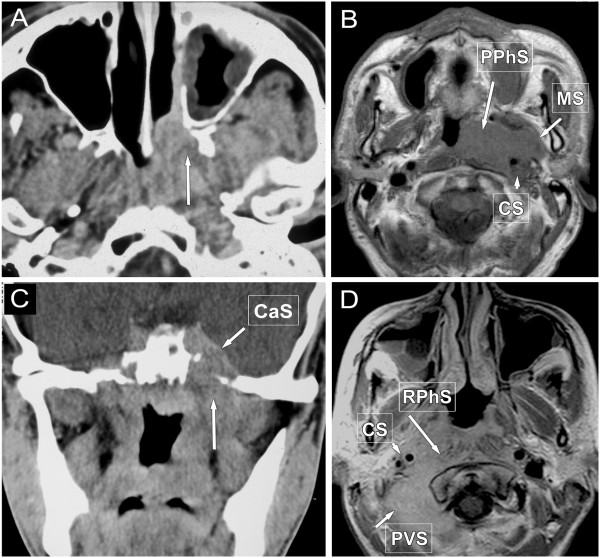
Different patterns of spread in nasopharyngeal cancer. A: Transverse post-contrast CT scan shows tumour destructing the left medial pterygoid lamina (long arrow), exceeding the choana and spreads along the lateral wall of the nasal cavity. Stage T3. B: contrast-enhanced T1-weighted MRI image demonstrates CA etxension to the parapharyngeal- (PPhS, long arrow), carotid- (CS, arrow-head) and masticator space (MS, short arrow) on the left side. Stage T4. C: Coronal contrast-enhanced CT image shows left sided destruction of the skull base (long arrow) and tumorous infiltration into the cavernous sinus (CaS, short arrow). Stage T4. D: Transverse post-contrast T1-weighted MR image demontrates tumour spread into the retropharyngeal space (RPhS, long arrow) on the right side, infiltrating the longus colli muscle, involving also the carotid- (CS, arrow-head), and paravertebral space (PVS, short arrow). Stage T4.
